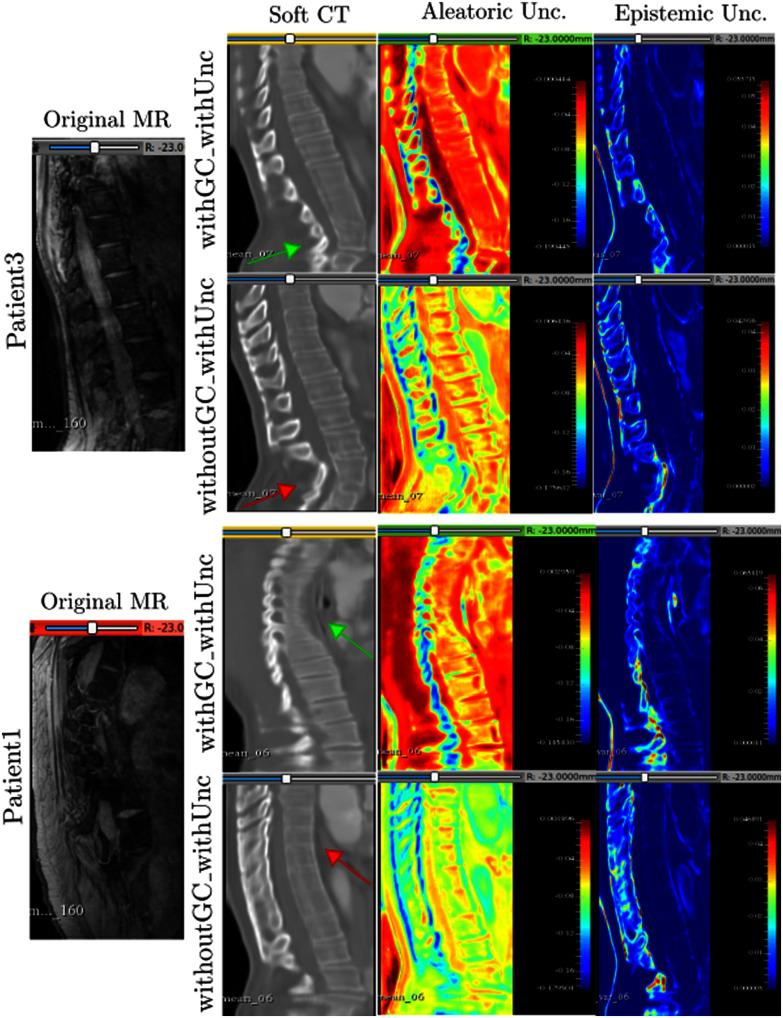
The soft translations along with aleatoric and epistemic uncertainties. Left-to-right: original sagittal MR slices for Patient1 and Patient3, “soft” CT predictions, aleatoric maps learned by the models, and epistemic uncertainties. Top-to-bottom: 1st and 3rd rows show results with GC, 2nd and 4th rows show results without GC. Green arrow points to the spinal curvature better translated with GC and red arrow shows the same region translated without GC. Blue and red regions in the uncertainty maps refer to low and high uncertainties respectively. “Unc.”$=$ Uncertainty.
